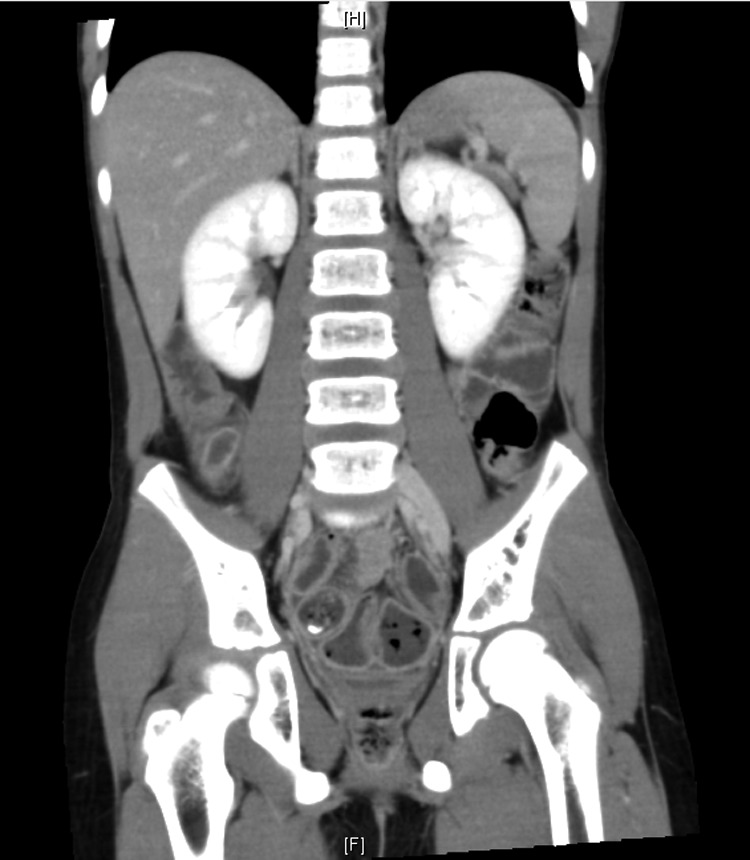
Abdominal CT revealed an engorged appendix with no hydronephrosis over the bilateral kidney.
